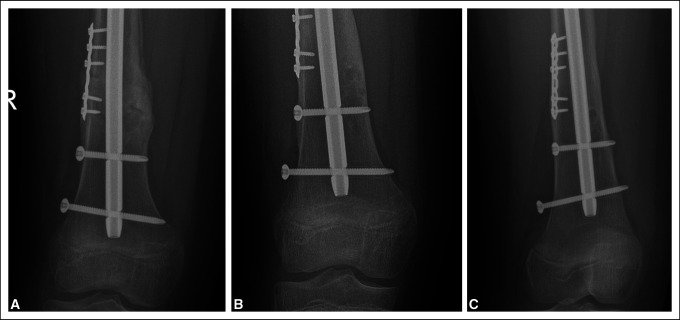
Radiographs showing evidence of implant loosening with haloing visualized around the distal tip of the nail and locking bolt. A, Not seen at 3 months. B, First noted at 7 months postoperatively with mild haloing around distal interlock. C, Valgus deformity seen in conjunction with a loosened distal interlocking screw.
